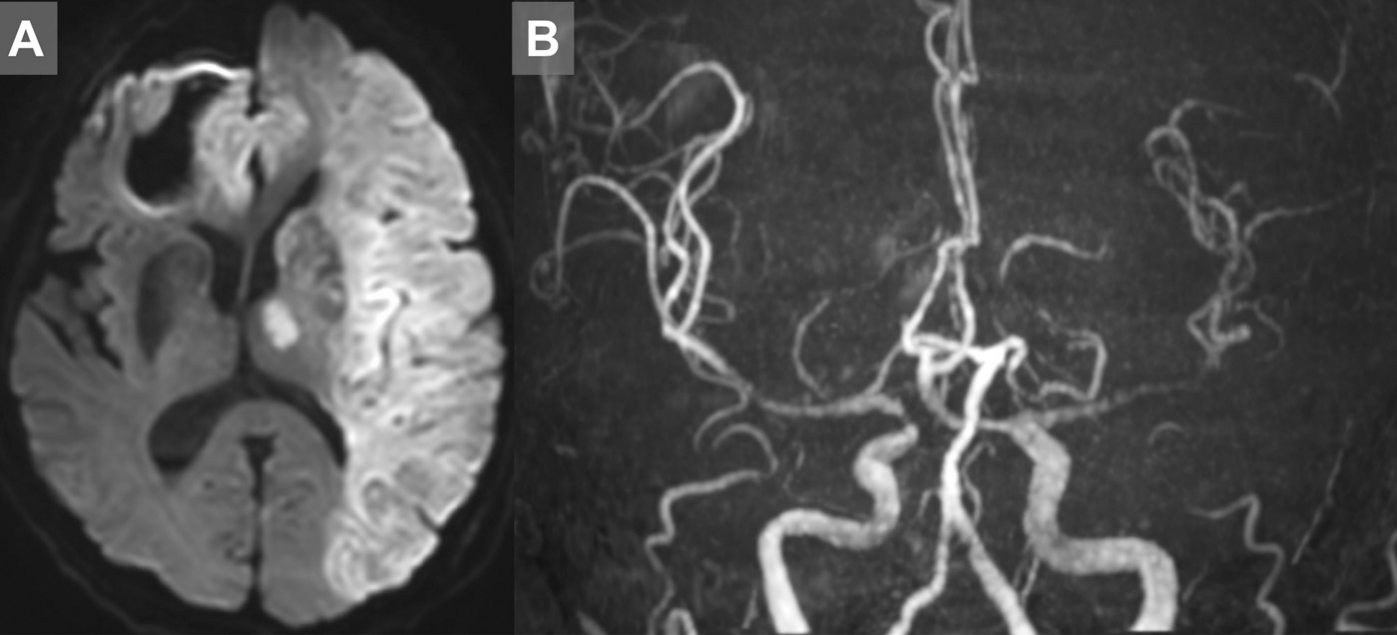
Postprocedural image (A and B) Magnetic resonance imaging performed on the day after the procedure, showing successful revascularization but extensive infarction.
